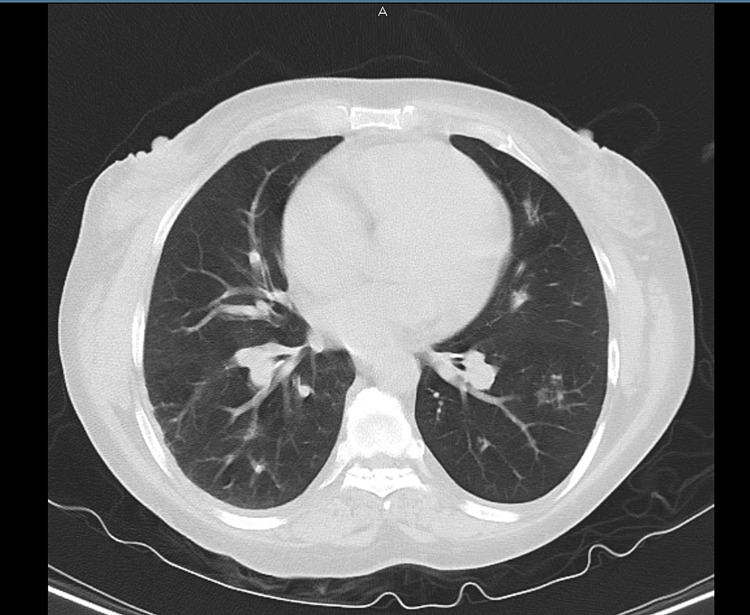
CT chest showing no consolidation and emphysematous changes due to COPD. Incidental finding of small GGO in the left lower lobe. CT: computed tomography; COPD: chronic obstructive pulmonary disease; GGO: ground-glass opacities
